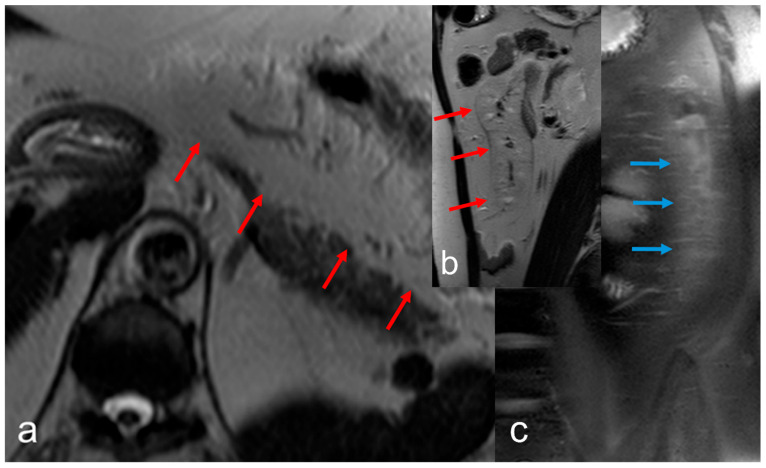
MRI findings in ulcerative colitis: (a) axial and (b) sagittal MRI showing MRI-defined MP with a pseudocapsule, embedded nodules/fat-ring configuration, and associated bowel-loop displacement/mass effect (red arrows); (c) coronal MRI showing inflammatory wall thickening (blue arrows) of the descending colon.
